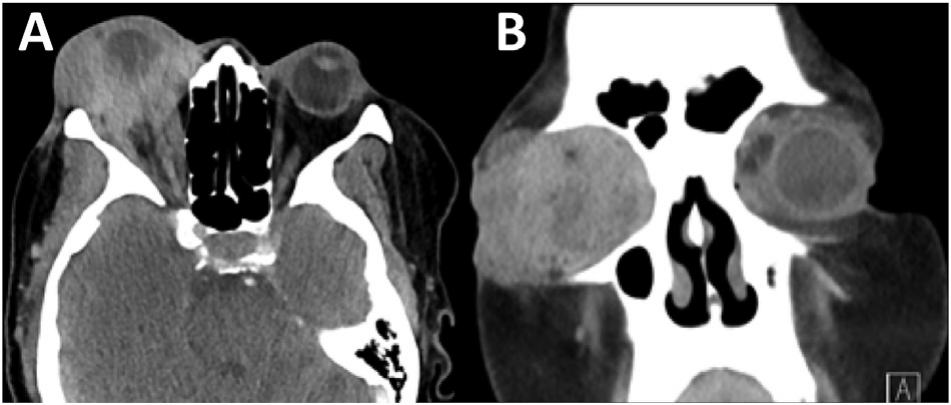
CT scan with contrast through mid-orbit. Axial (A) and coronal (B) views demonstrate a large heterogeneously enhancing mass within the right orbit, involving pre- and post-septal soft tissues. The lesion is inseparable from the lacrimal gland and encases the globe resulting in severe scleral deformation consistent with globe rupture.
